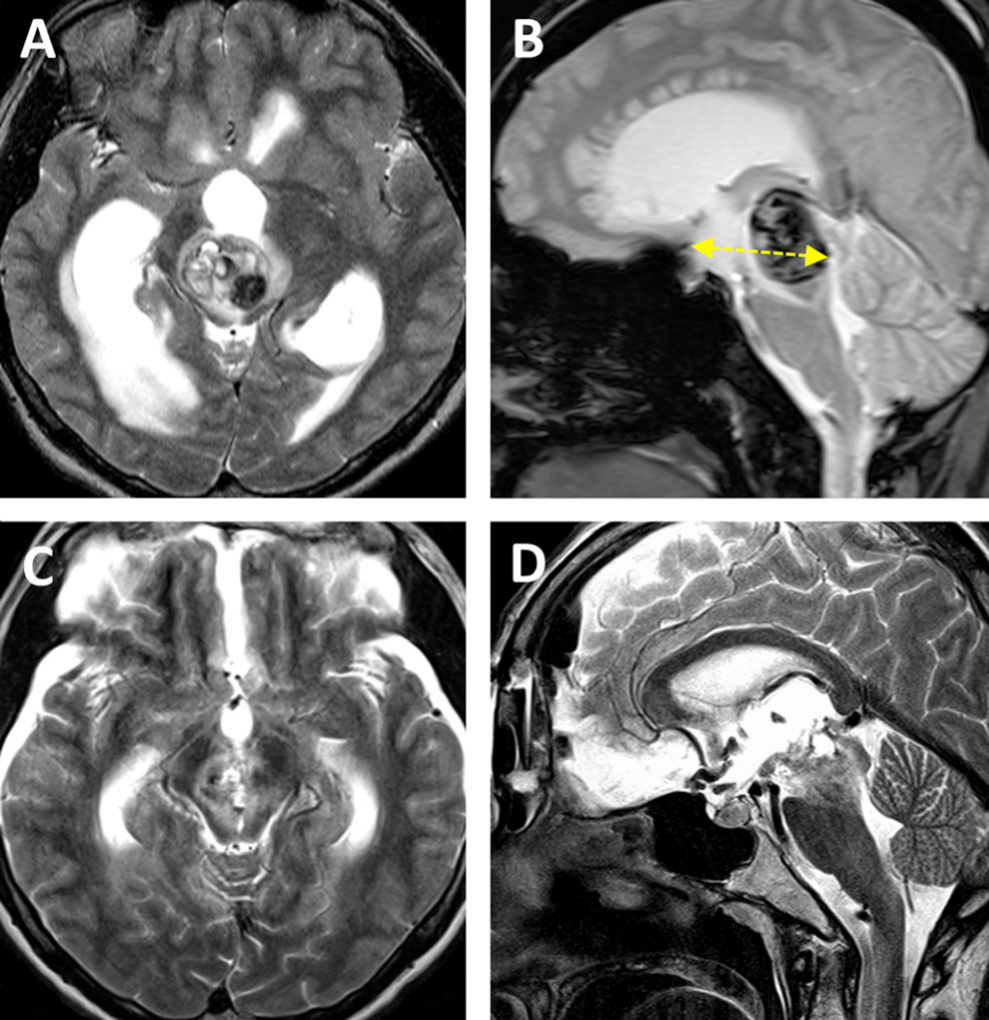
Pre- and postoperative MRI of case 9. a, b Preoperative MRI of a hemorrhagic midbrain cavernoma that was classified as “central lesion.” The yellow dotted line shows the tumor depth (42.5 mm). c, d Postoperative MRI after total removal of the vascular lesion
